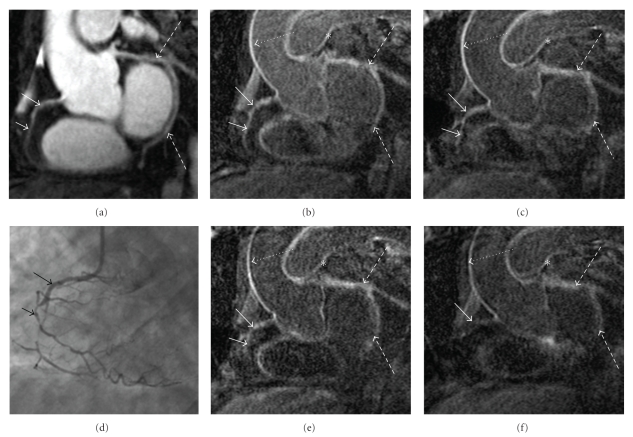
(a) MR-coronary angiography of the RCA (white arrows) showing intraluminal signal loss at medial and distal RCA as demonstrated by coronary angiography (d) (black arrows). Reformatted inversion recovery MR images: (b) 33 minutes; (c) age range 50 minutes; (e) 68 minutes, and (f) 77 minutes post contrast administration demonstrate strong enhancement of the coronary vessel wall at the earliest time-points and signal loss over the time-intervals (white arrows). Note strong enhancement of the vessel wall of the aortic arch (dashed arrows) in contrast to the loss of enhancement of the RCA (solid arrows) and LCX (interrupted arrows) over time. There is also enhancement of the pulmonary artery (asterisk).
